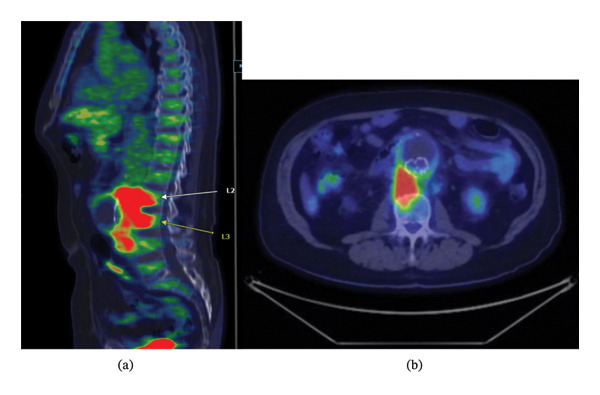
F‐18 FDG PET/CT skull to thigh. Sagittal view (a) and transversal view (b).
